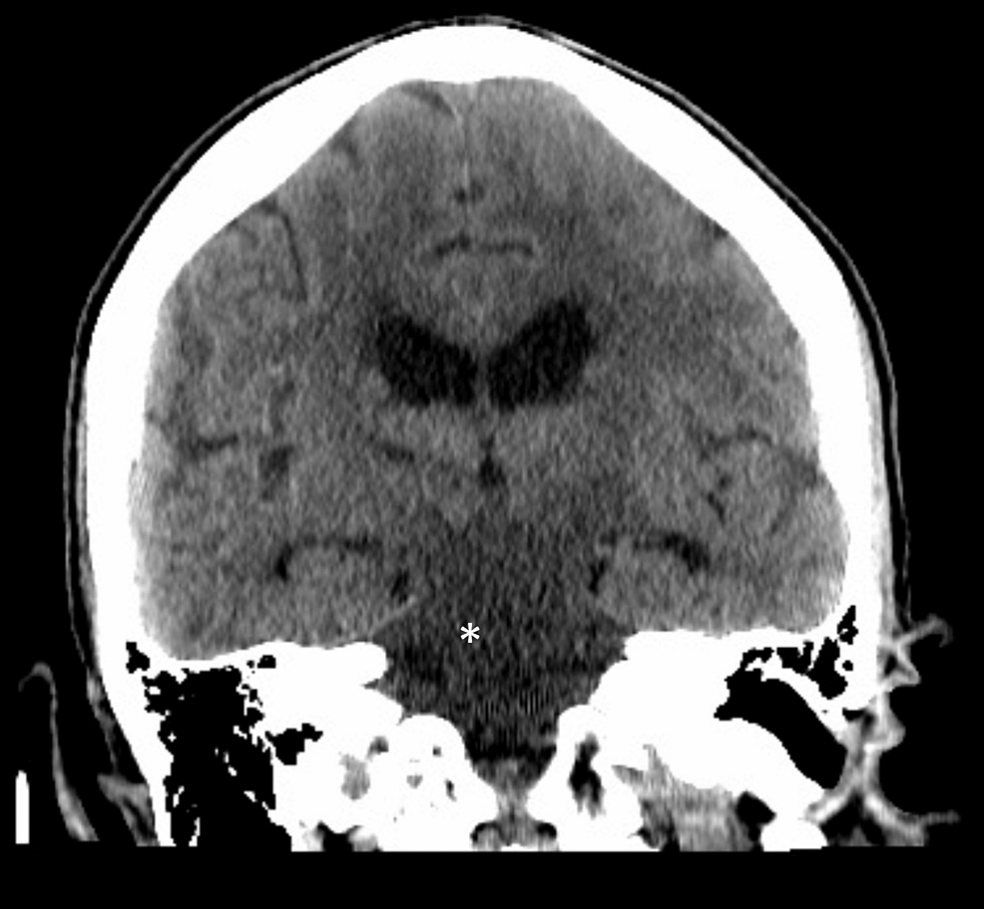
CT head coronal view done four hours after last known normal with hypodensity diffusely involving the midbrain, pons, cerebellar peduncles, and medulla (asterisk).
